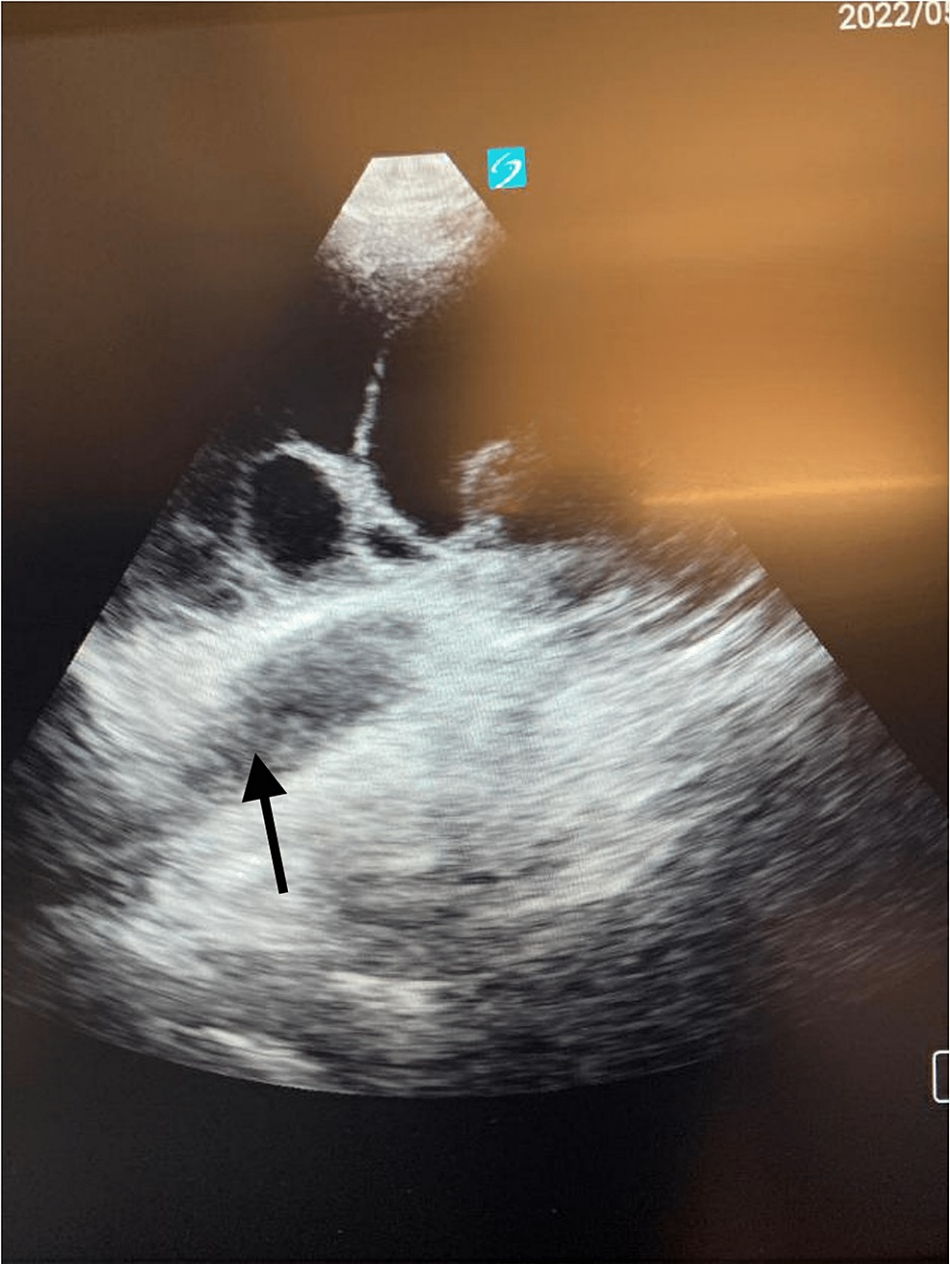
Transthoracic Echocardiography A transthoracic echocardiography showing fluid collection adjacent to the heart suggests pleural effusion (arrow).
